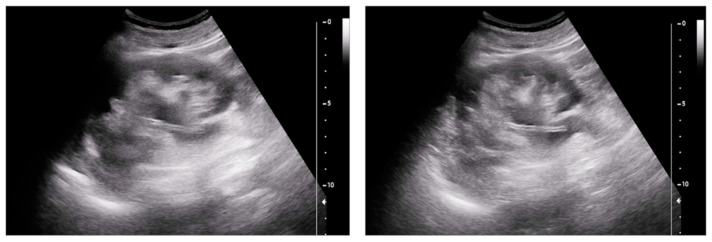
A nephrostomy catheter. On ultrasound, the stent can be readily visualized as a hyper-echoic structure located in the renal pelvis. Note how it is highlighted by the presence of a moderate ureter–pelvis distension.
